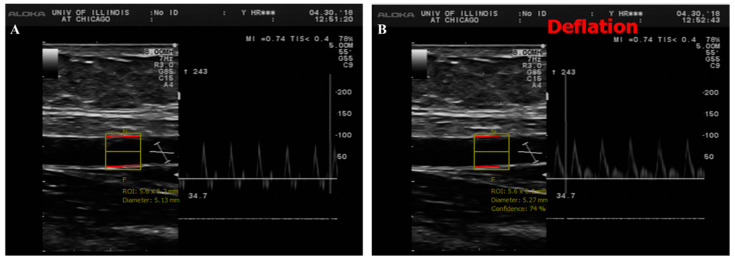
Duplex B-mode/pulsed wave Doppler (PWD) ultrasound of brachial artery flow-mediated dilation (FMD). This figure shows a long axis scan of brachial artery with simultaneous blood velocity profile by pulsed wave Doppler before (A) and after cuff deflation (B). For offline image analyses, a representative section of brachial artery is selected for an automated measurement of diameter. Baseline diameter (BSL) was averaged from a serial of recorded frames before cuff deflation and the maximum diameter was measured after cuff deflation during reactive hyperemia (RH).
